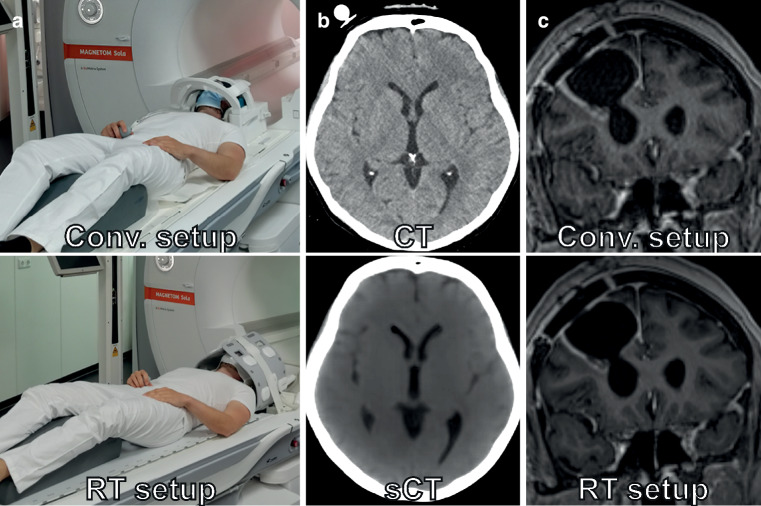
SRT treatment setup with mask immobilization. a Conventional imaging setup in diagnostic head coil (*top*) vs. imaging setup in RT treatment position with mask immobilization enabled by flexible receiver coils (*bottom*). b Conventional planning CT (*top*) vs. Synthetic CT (sCT) reconstructed from MRI sequences (*bottom*). c Less motion artifacts with mask immobilization in the RT imaging setup (*bottom*) compared to the standard imaging setup without mask immobilization (*top*)
